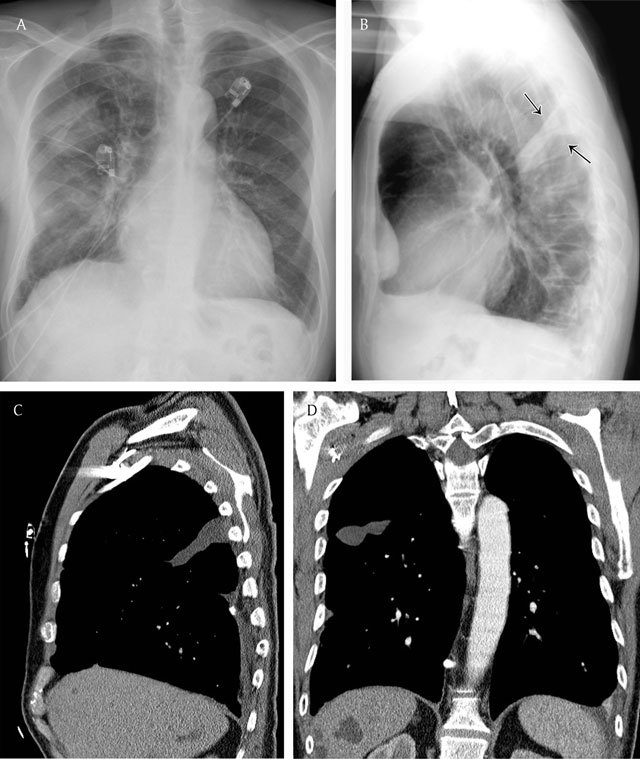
Lenticular configuration of loculated fluid in the fissure – Frontal chest radiograph (a) shows a hazy increase in opacity, not sharply defined in the upper part of the right hemithorax. Lateral chest radiograph (b) shows the loculated fluid in the major fissure (black arrows), confirmed on sagittal (c) and coronal (d) reformatted images on CT.
